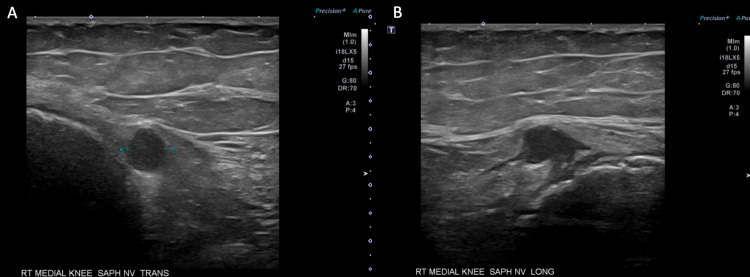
Ultrasound images showing a neuroma of the patellar branch of the right saphenous nerve adjacent to the knee. A: Transverse view. B: Longitudinal view.
